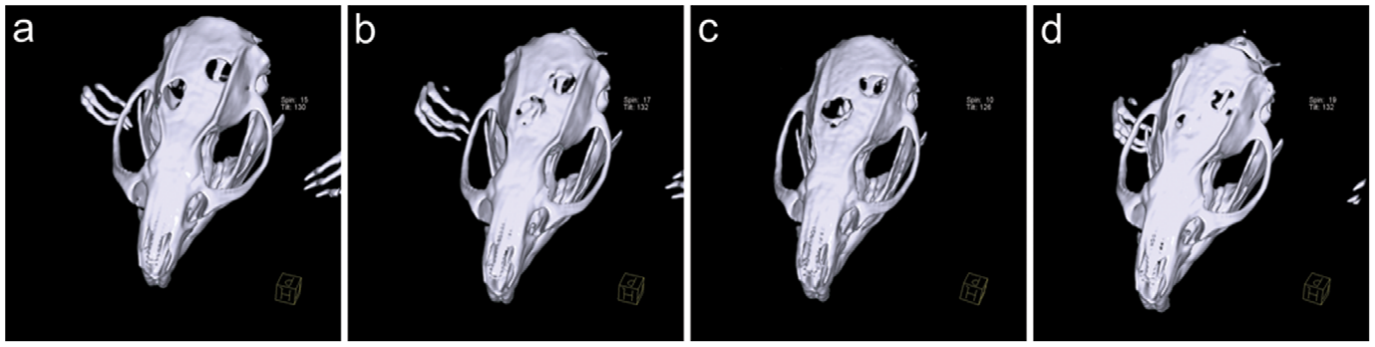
3D reconstructions of cranial CT-scans used for quantification of radiological bone density. (a) Negative control, 12 weeks. (b) Positive control, 12 weeks. (c) PLLA, 12 weeks. (d) PLLA/BMP-2, 12 weeks.
